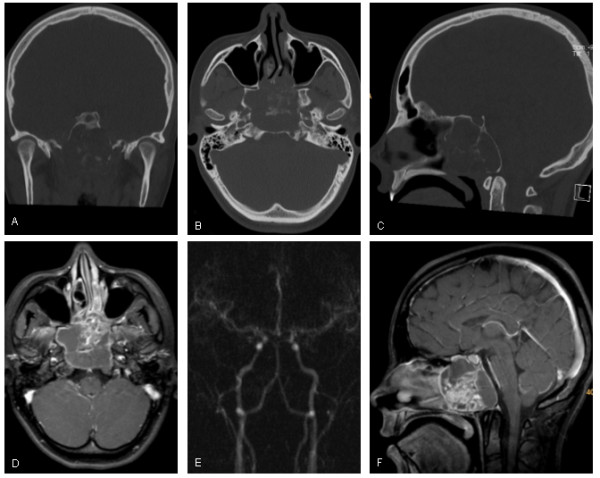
Extracranial craniopharyngeoma. A-C: Coronal, axial and sagittal reconstructions of a spiral CT scan. Note the expansively growing mass with calcifications, thinning and remodeling of the walls of the pterygoid processes, predominantly on the right side, and the anterior parts of the clivus with preserved posterior margin. D and F: Axial and sagittal contrast-enhanced T1-weighted images from a 3D data revealing a partly solid and cystic mass of the clivus and sphenoid cavity with expansion into the ventral the ethmoid sinuses and the nasal cavity, caudally limited by the plane of soft and hard palate and cranially by the sella. E: Early arterial phase of a time resolved contrast-enhanced MR angiography to exclude an juvenile angiofibroma. Neither pathological arterial vessels nor arteriovenous shunts were visible.
